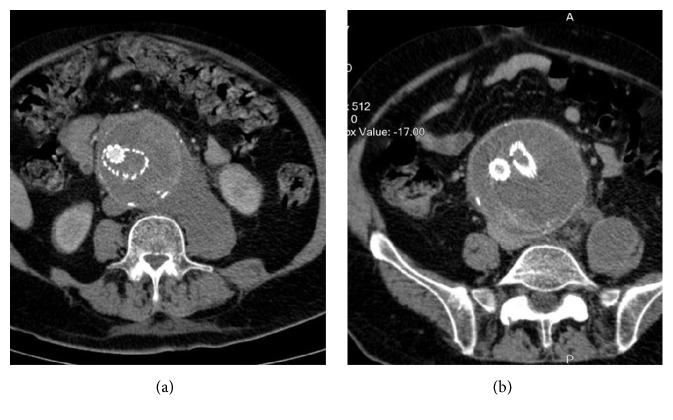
(a) Urgent CT reveals rupture of the aneurysm sac (a) in the arterial phase and (b) in the delayed phase without obvious endoleak.
